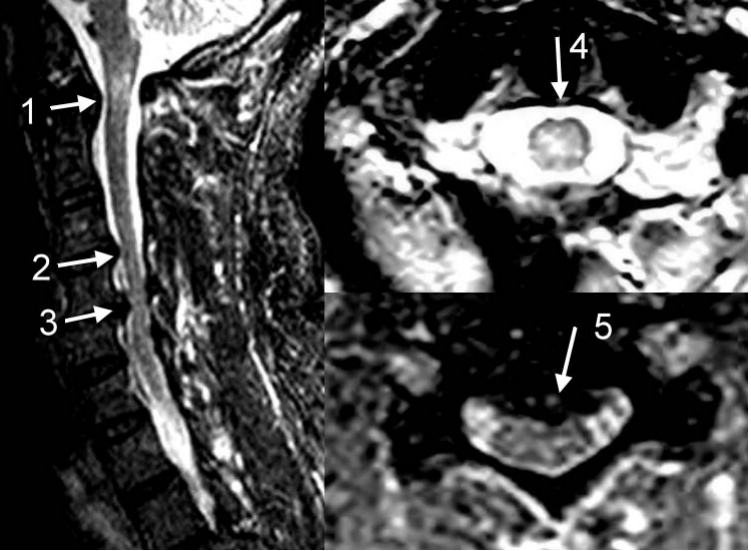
T2 image of Cervical spine MRI. Arrow 1: Hyper dense lesion at C1 level. Arrow 2 and 3: Bulged disc over C4-5, C5-6 with spinal cord compression. Arrow 4: Cross section of C1 level. The hyper dense lesion distributes over the central area and bilateral lateral column. Arrow 5: Bulged disc of the C5-6 with cord compression in the anterior aspect
